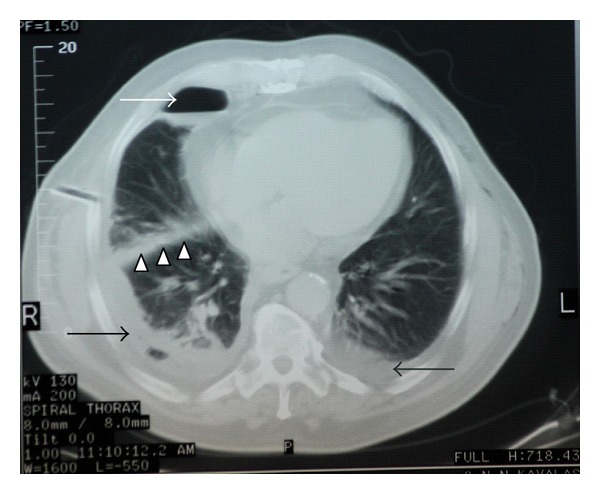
Computed tomography of the thorax at day 13 demonstrates right pneumothorax (white arrow) with lung infiltrations and bilateral pleural effusions (black arrows). The chest tube is visible (head arrows).
